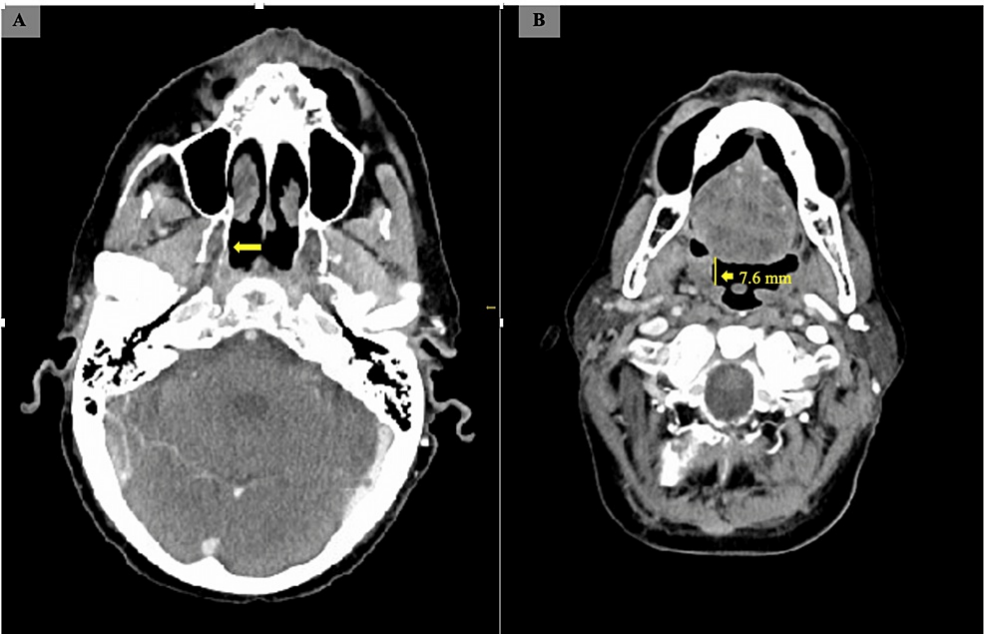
Post-treatment computed tomography of the head and neck with contrast Post-treatment computed tomography of the head and neck with contrast (palatine tonsil): (A) Resolution of lesions at right masticator space and pterygoid muscle, (B) Decreasing nodule size of about 50% at the right palatine tonsil.
